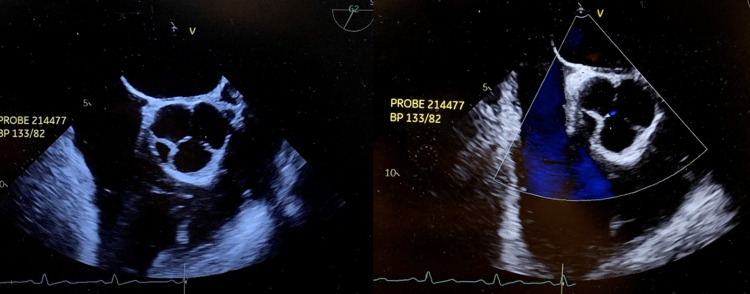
Quadriscuspid aortic valve on transesophageal echocardiogram (TEE) short axis view (right and left).
